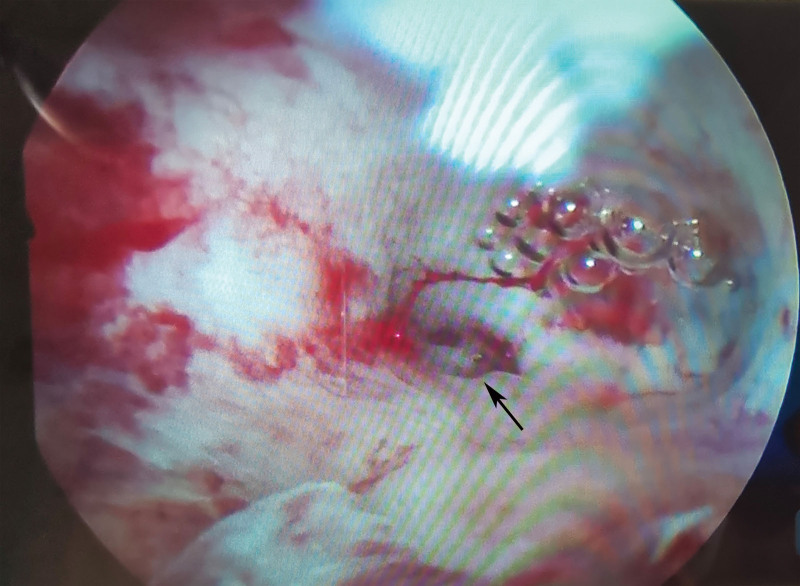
Hysteroscopy revealing a uterine sinus tract (3 × 3 × 3 mm) (arrow)in the left posterior wall of uterus near the corner 4 months later after HIFU. HIFU = high-intensity focused ultrasound.
